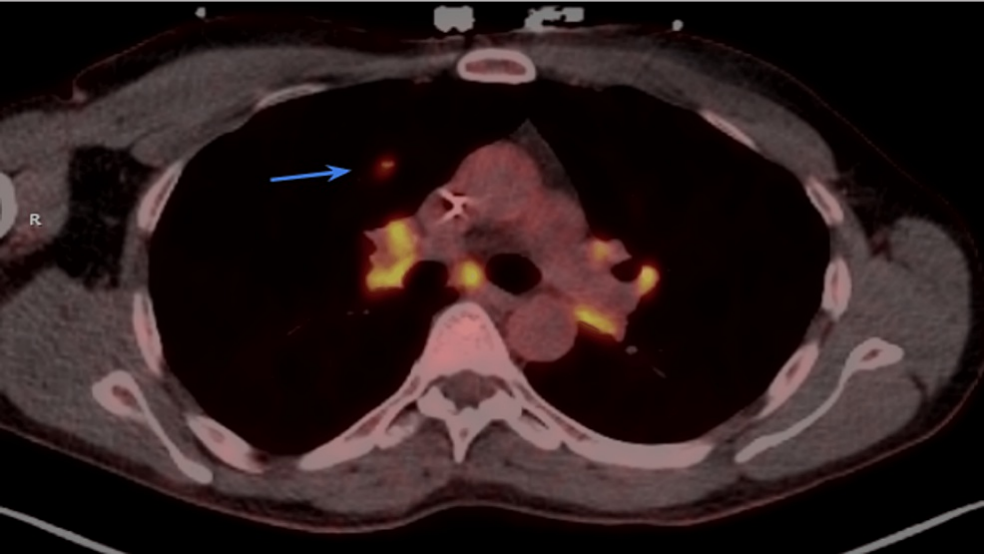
PET-CT chest of hypermetabolic activity in a pulmonary nodule, suggestive of infiltrative disease like sarcoidosis. PET-CT: positron emission tomography-computed tomography
